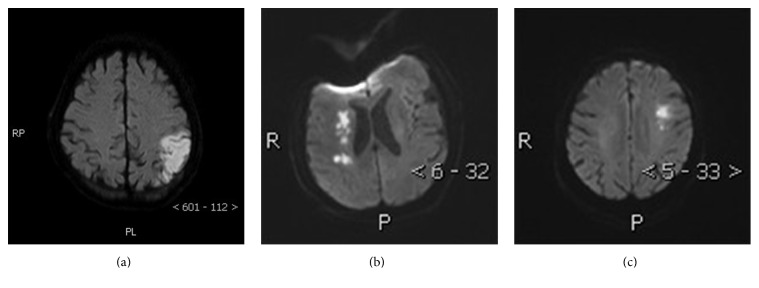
Typical examples of cerebral watershed infarcts in patients with carotid artery stenosis. Diffusion weighted imaging (DWI) showing (a) cortical watershed infarction between the posterior cerebral artery (PCA) and middle cerebral artery (MCA) cortical territories, (b) right-hemisphere internal watershed infarction on diffusion weighted imaging, and (c) cortical watershed infarction between the anterior cerebral artery (ACA) and MCA cortical territories.
